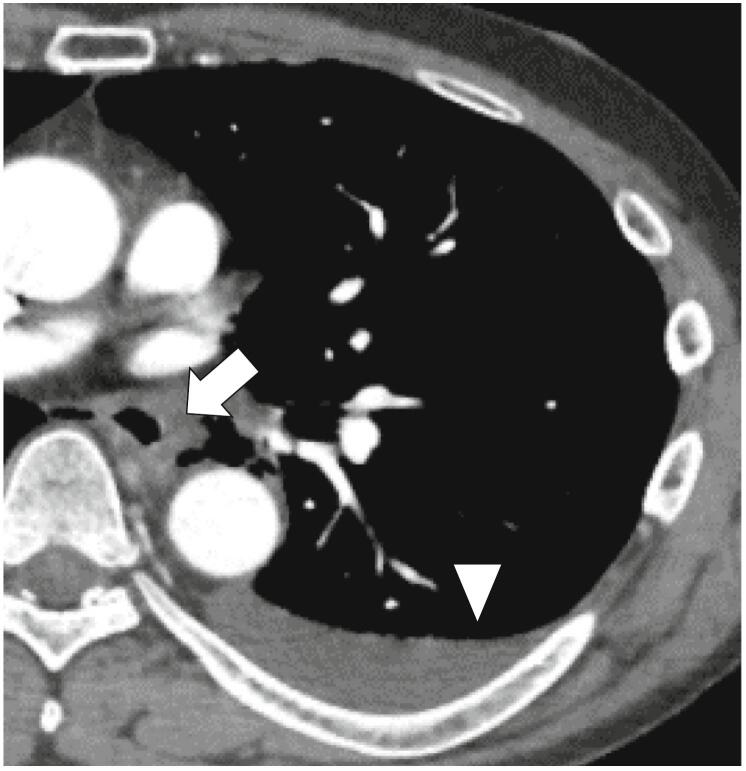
A chest CT showing the mediastinal fluid (arrow) and left pleural effusion (arrowhead).
